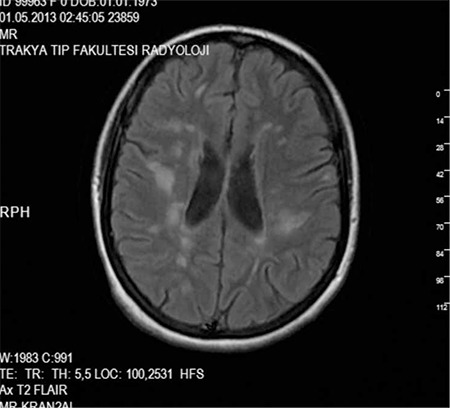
Cranial magnetic resonance imaging showing multifocal demyelinating plaques in the periventricular area
